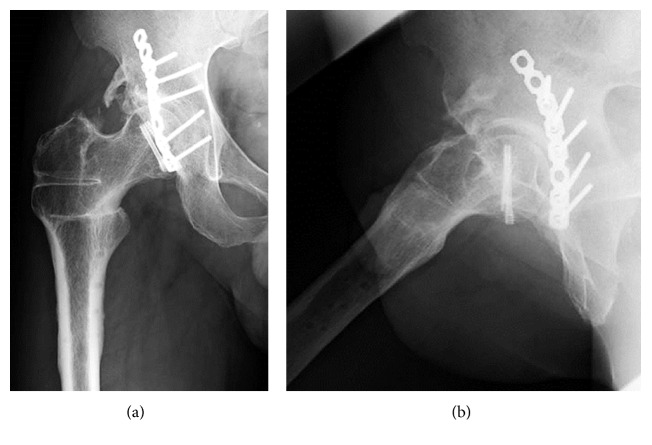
Case 1. (a) Anteroposterior X-ray image of the right hip at 3 years after osteotomy. (b) Lateral X-ray image of the right hip at 3 years after osteotomy.
